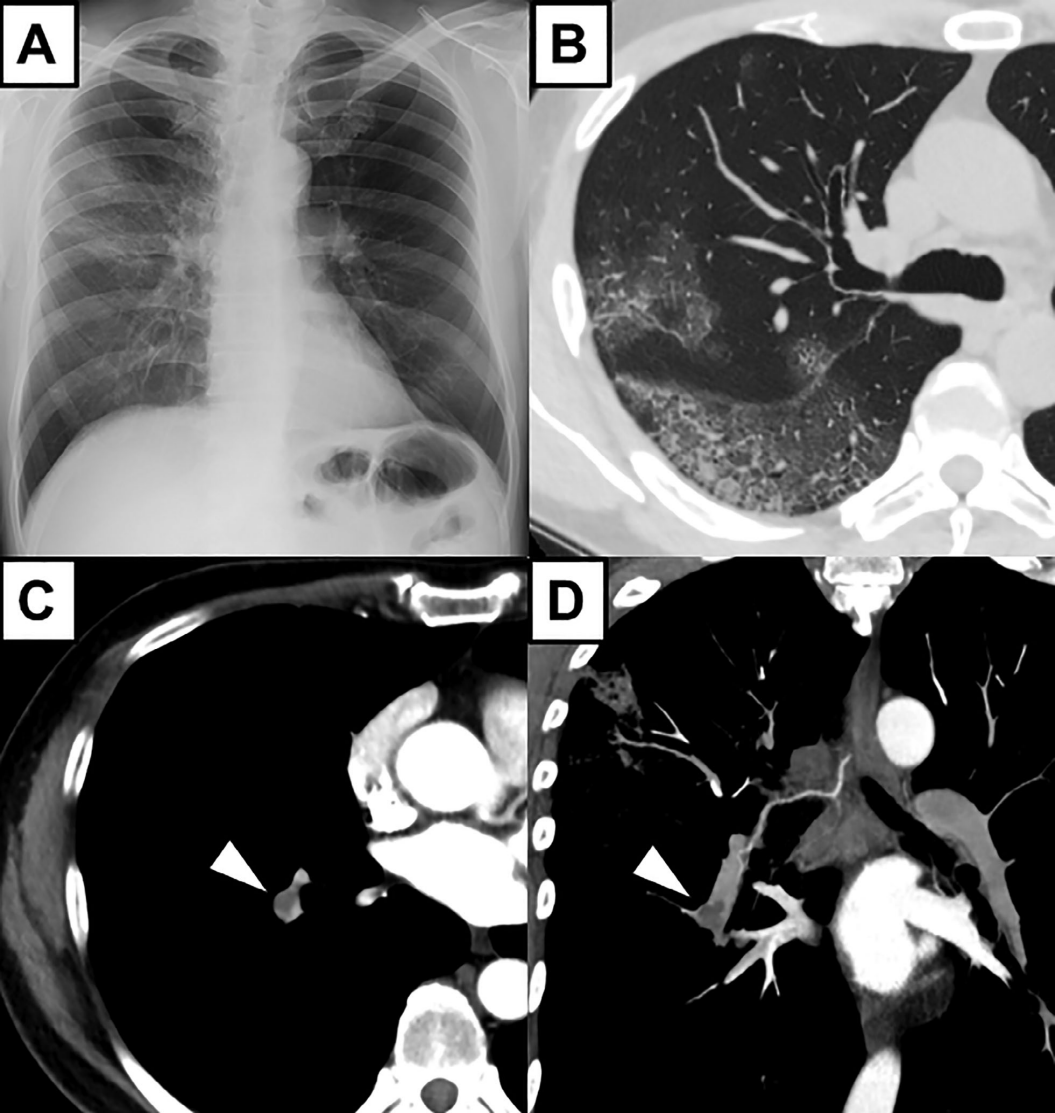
(A) Chest X‐ray on admission. Ground‐glass opacity (GGO) was observed in the right lower and middle lung field. (B) Chest computed tomography (CT) on admission showed GGO in the upper lobe and S6 region of the right lung. (C, D) Chest contrast CT on day 5 showed poor contrast enhancement in the right pulmonary artery, suggesting pulmonary embolism (PE) (white arrowheads).
